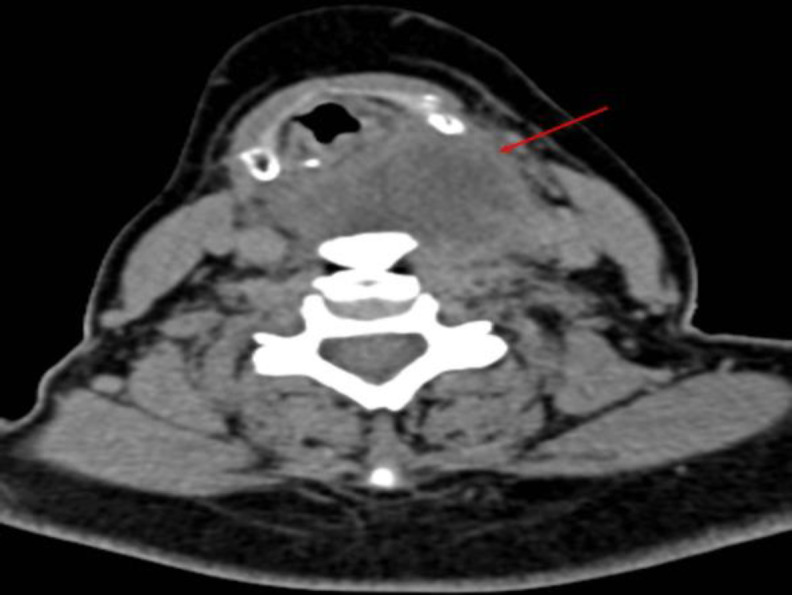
Neck CT (axial scan) showing suppurated diverticulum at the level of C6-C7 (red arrow) and DISH C6-T1 level
